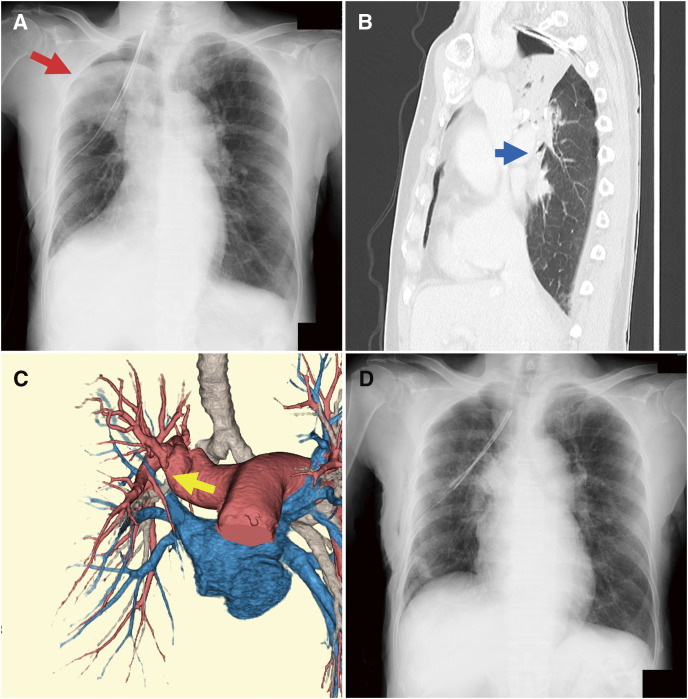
(A) Postoperative chest radiograph showing an infiltration shadow (red arrow) in the right upper lung field. (B) Postoperative sagittal CT scan showing interruption of the middle lobe bronchus (blue arrow) with atelectasis. (C) Three-dimensional CT scan showing blurring of the middle lobe bronchus (yellow arrow). (D) A chest radiograph on postoperative day 1 showing good extension of the lungs. CT: computed tomography
